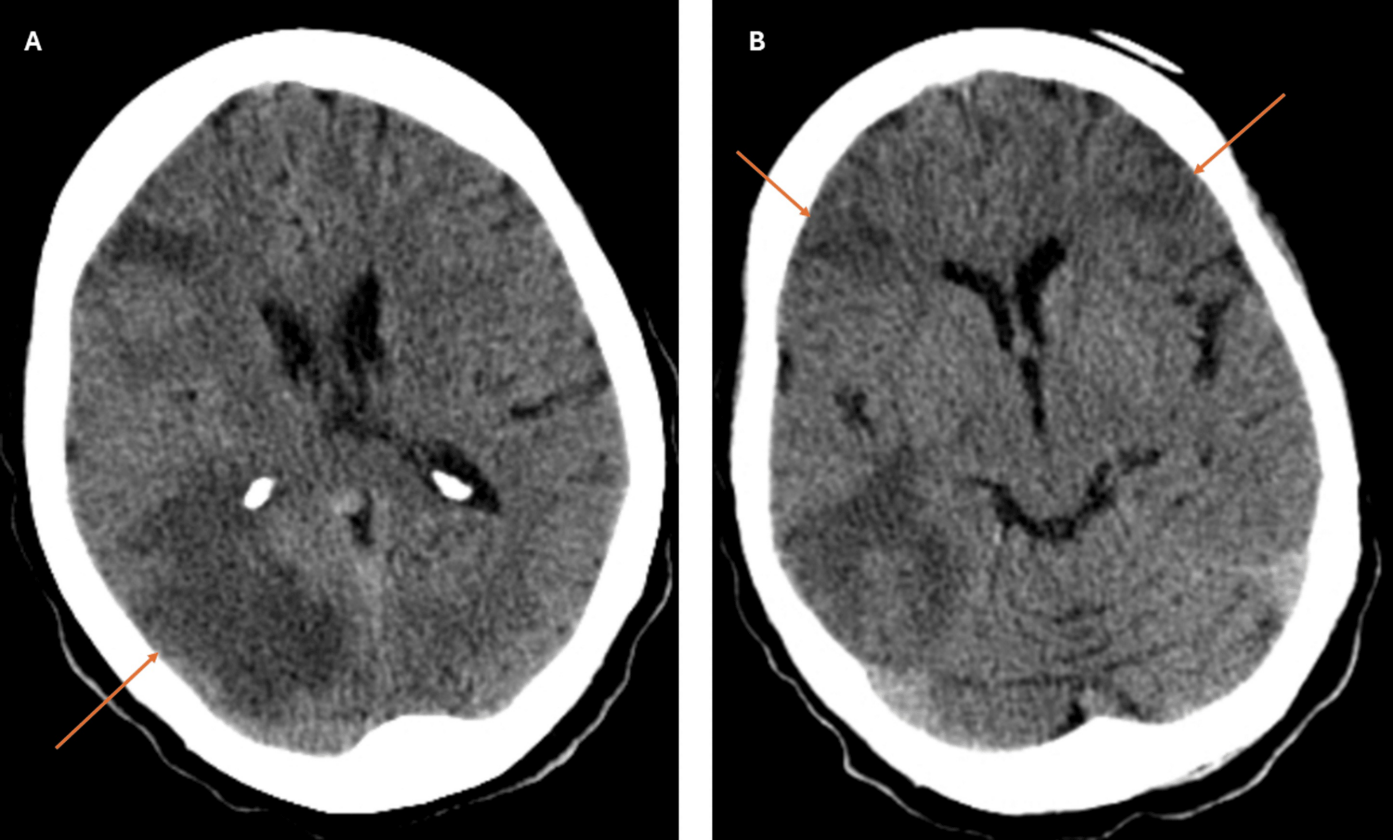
Images of brain CT scan Brain CT scan at hospital admission showing a hypodense lesion involving the right parieto-occipital region (arrow) with a predominant cortico-subcortical edematous component, associated with local sulcal effacement and right lateral ventricle collapse (A). Additional smaller lesions with edematous components (arrows) were noted bilaterally in the frontal regions (B).
